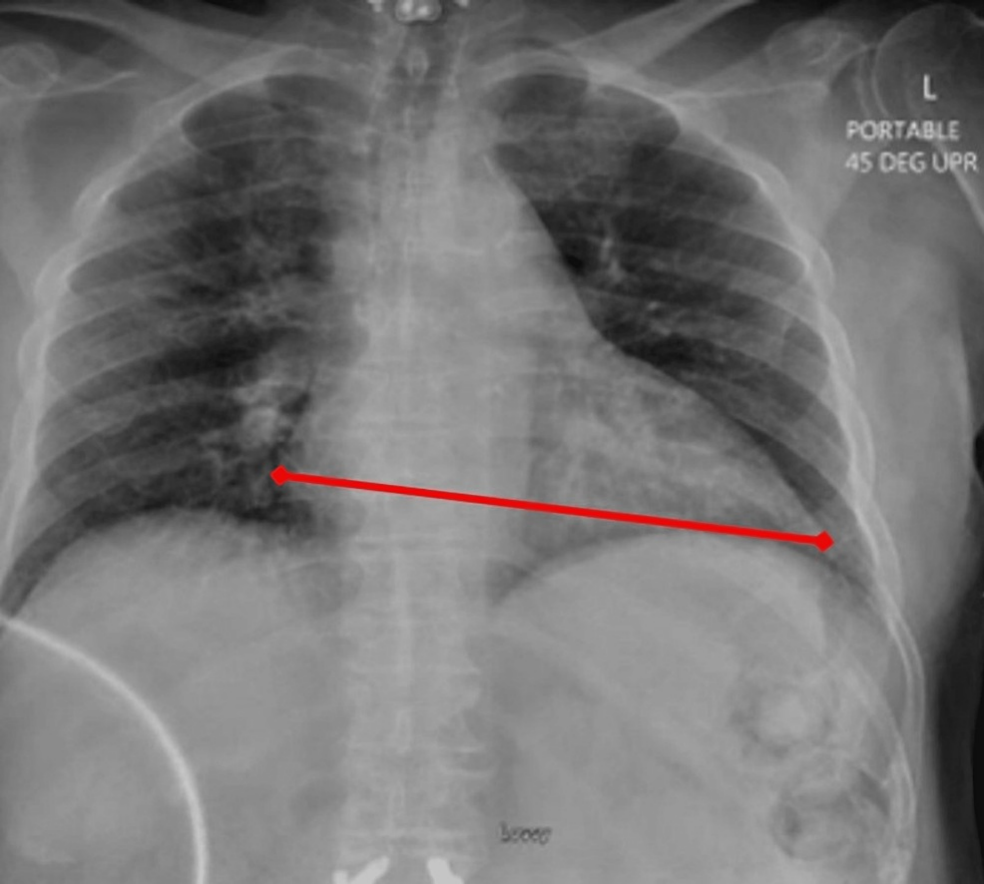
Chest radiograph (anteroposterior view) - hospital day two Cardiomegaly (red line) and diffuse interstitial coarsening are visualized on the chest radiograph.
